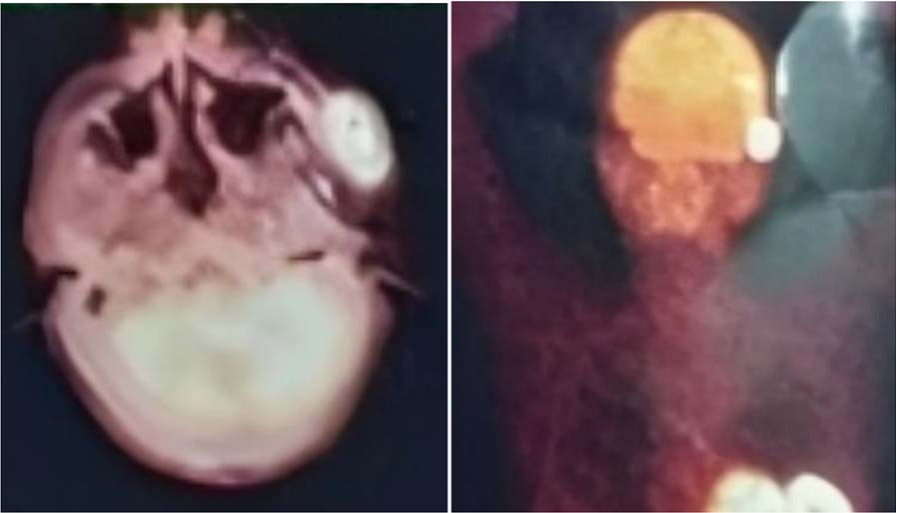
PET-CT demonstrating an unclear, heterogeneous mass that leachates into the skin and adipose tissue underneath it and the left masticatory muscle measuring 40 × 20 mm showing pathological metabolic activity.
